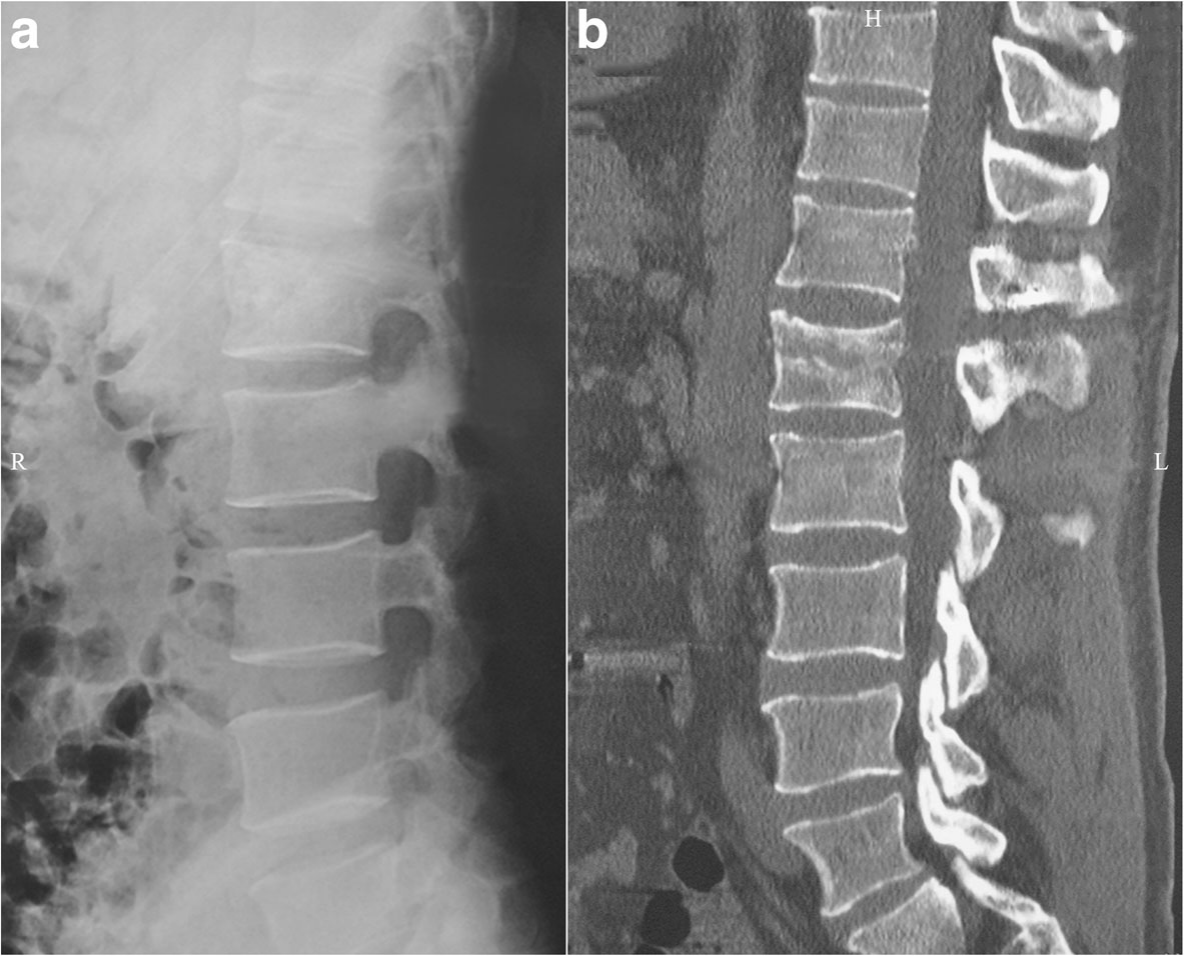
a Lateral radiograph of the patient shown in Fig. 2 at 2 years postoperatively. b CT of the patient shown in Fig. 2 at 2 years postoperatively
